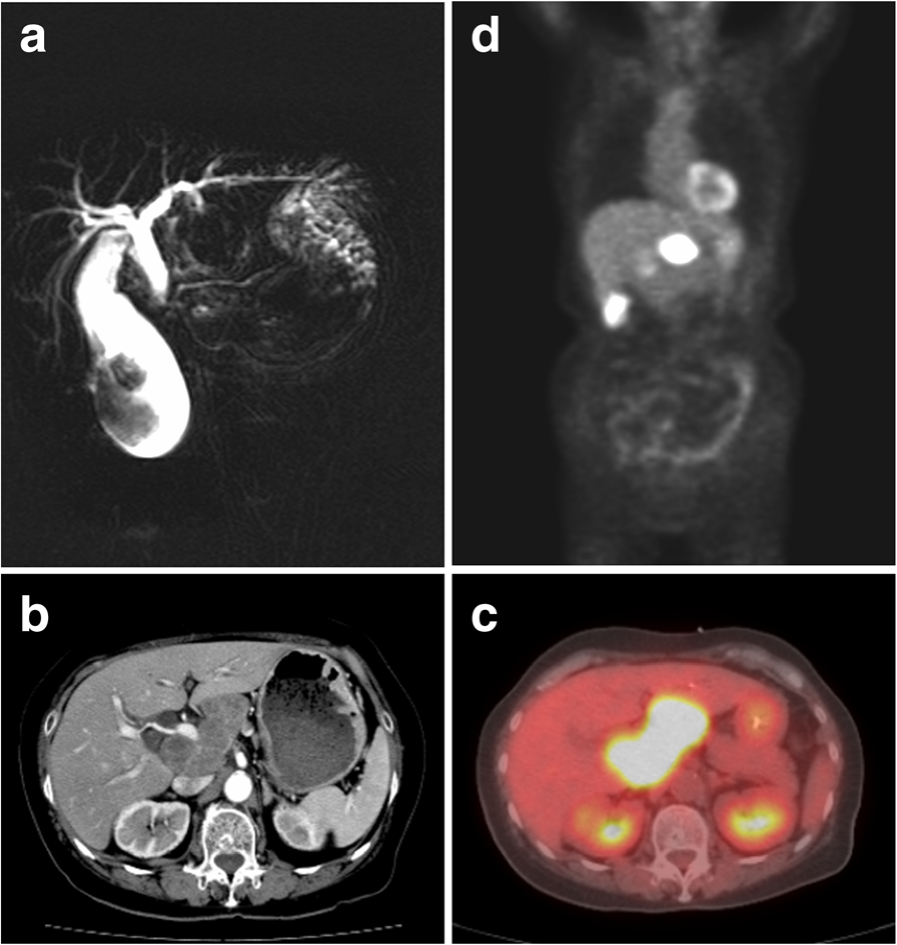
Preoperative imaging studies. Magnetic resonance cholangiopancreatography (MRCP) (a) and contrast-enhanced abdominal computed tomography (CT) scan (b) show a mass in a gallbladder with bulky hepatic lymph nodes surrounding the hepatic hilum. c, d Positron emission tomography/computed tomography (PET/CT) shows abnormal FDG uptake in the gallbladder (SUVmax 7.8) and the lymph node (SUVmax 13.4). No other metastases were identified
